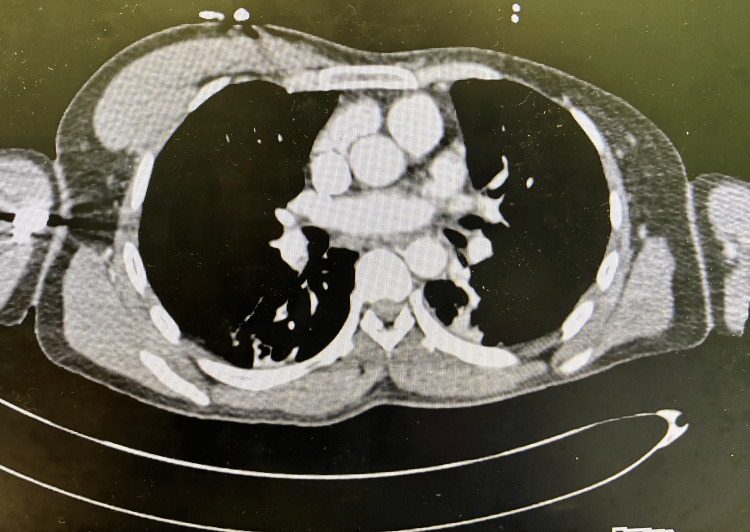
CT scan of the chest confirming the absence of left pectoral major and minor muscles
